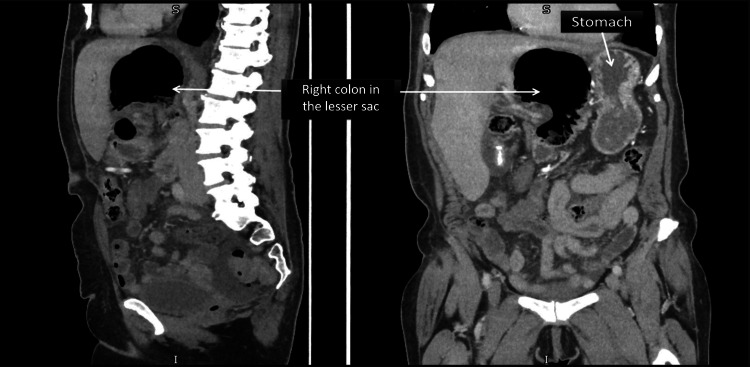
Sagittal (left) and coronal (right) CT views demonstrating the right colon within the lesser sac
